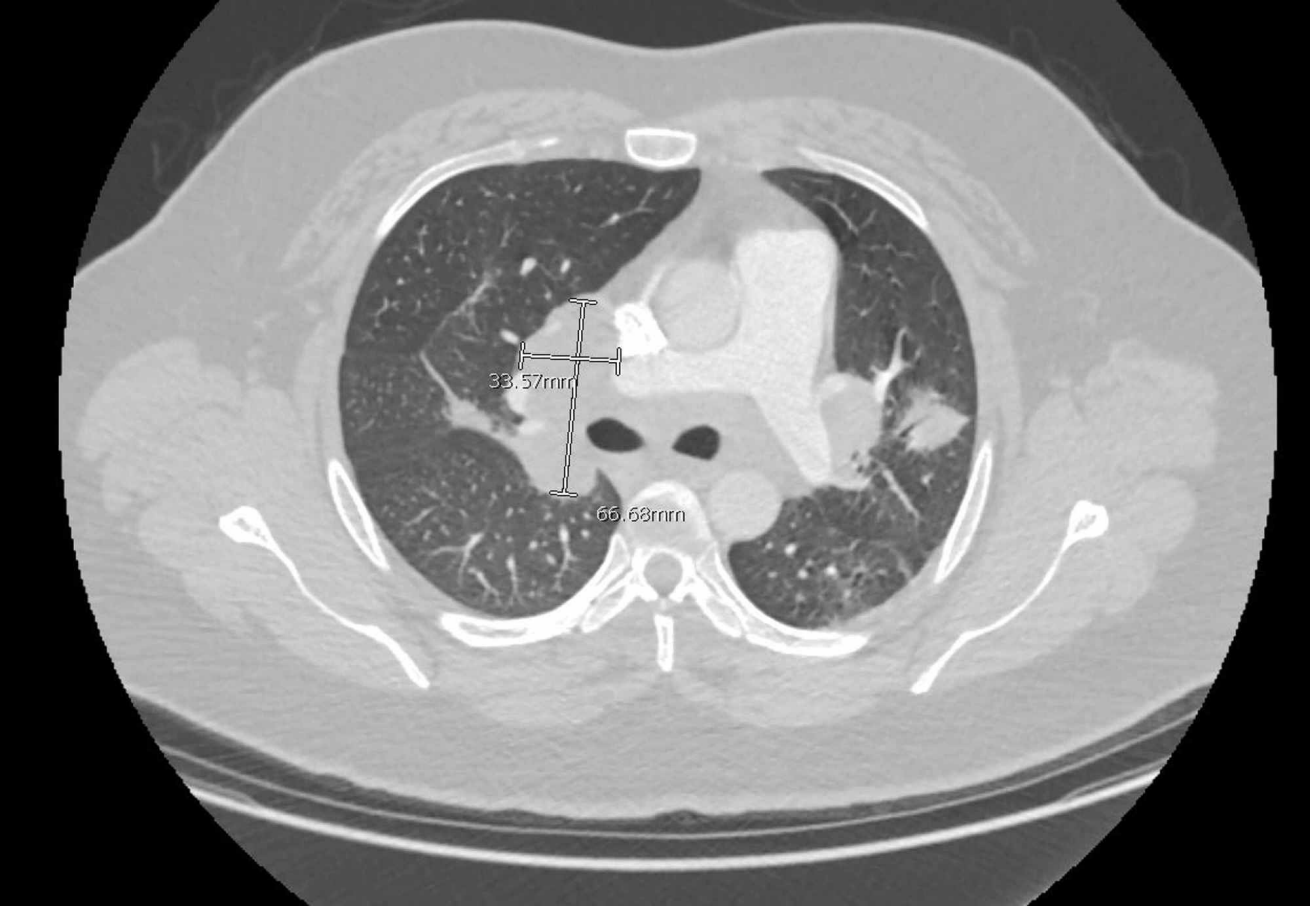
CT angiography chest transverse view showing extensively enlarged pathological hilar lymph nodes measuring 3.4 cm × 6.7 cm (dimensions marked in white).
